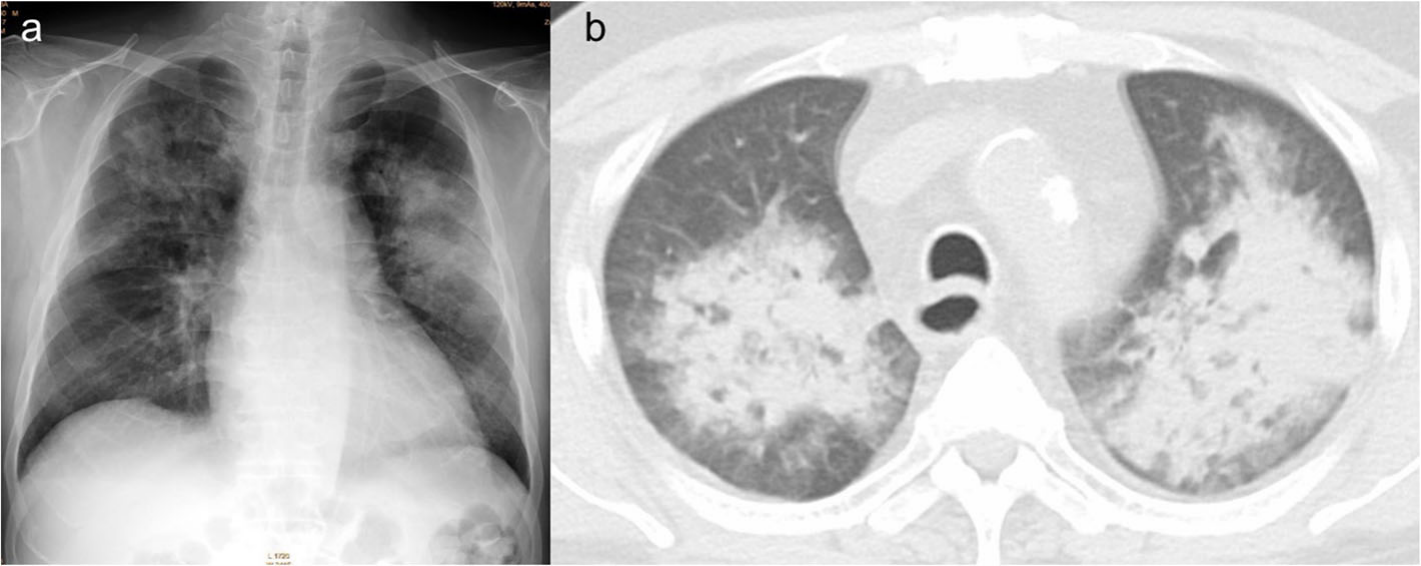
a Plain chest radiography showing a bilateral infiltrative shadow. b Computed tomography of the chest showing a bilateral infiltrative shadow in the hilar region dominant
